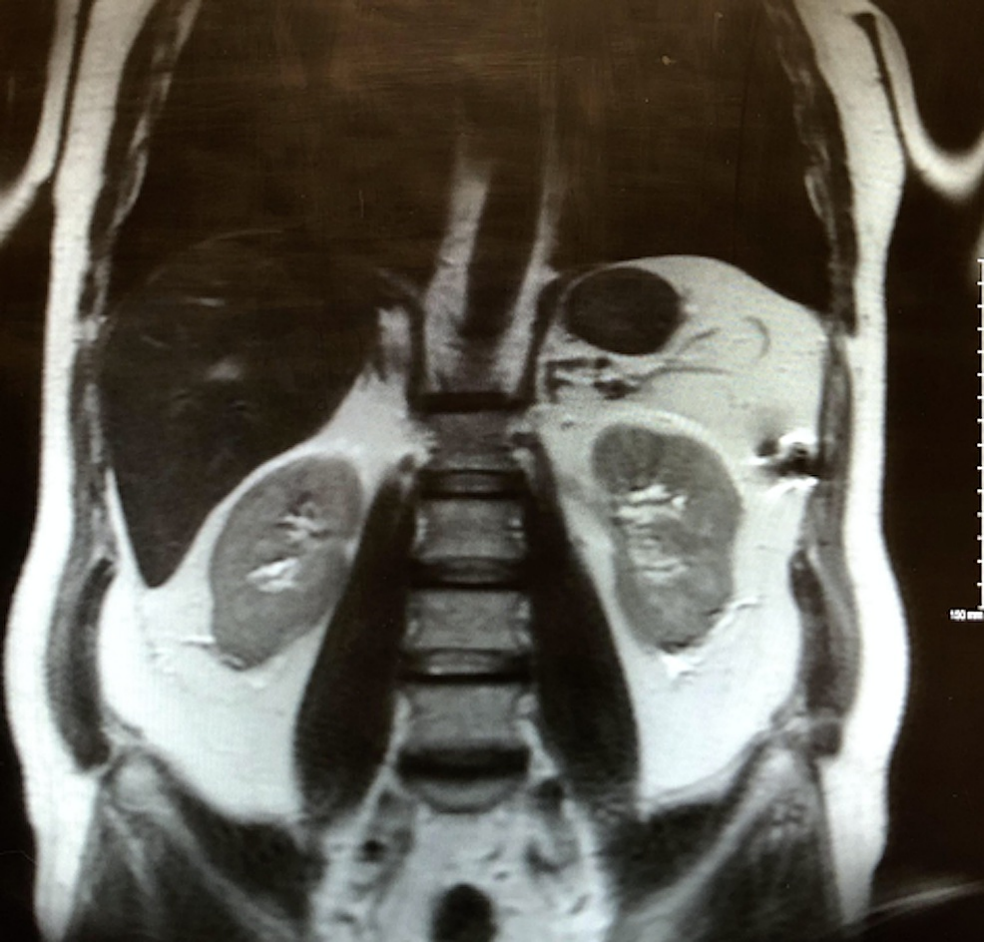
MRI abdomen showing no evidence for renal cell carcinoma
